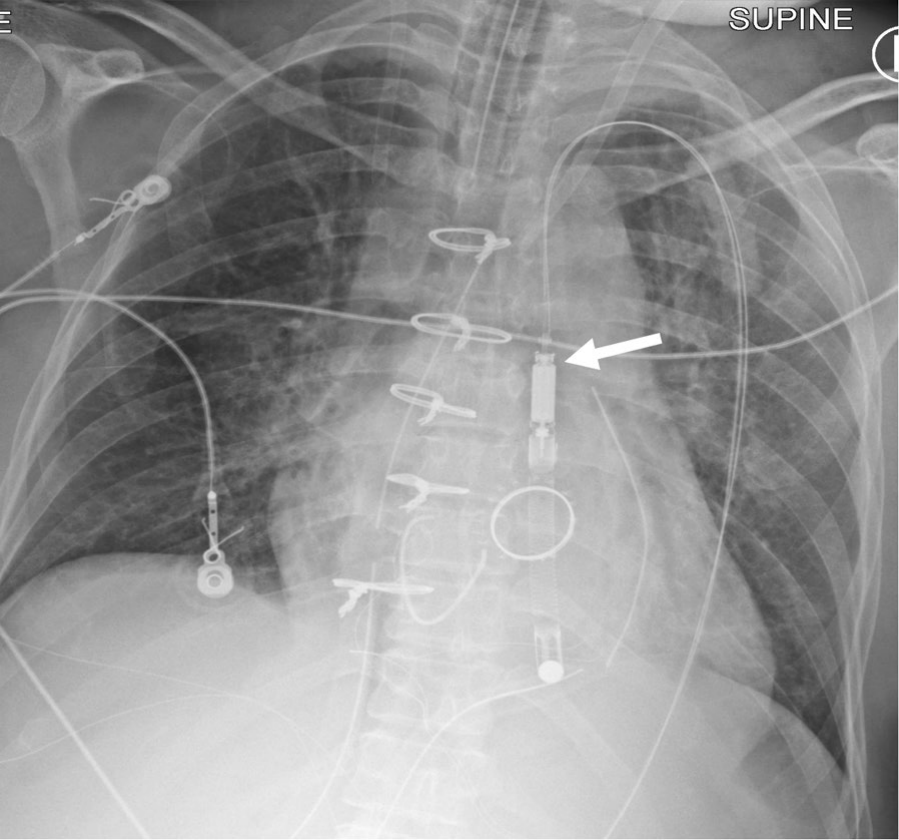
Chest radiograph demonstrating a right-sided placement of a LV Impella 5.5. This device was placed intraoperatively through a pulmonary artery graft. The inflow is located in the right ventricle and the outflow is located in the main pulmonary artery (white arrow) through the graft. The inlet and outlet directions can be changed depending on need to optimize flow. *Note*. There is no pigtail portion in the Impella 5.5 which helps differentiate this device. (LV—Left ventricle)
